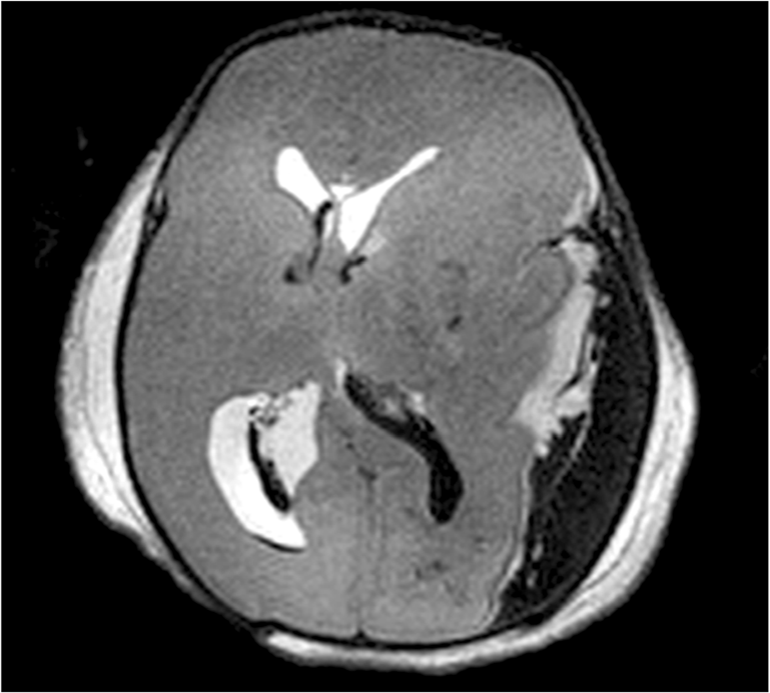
PMMR to document injuries. Post mortem imaging is useful to document the extent of intracranial injury prior to autopsy. In this case, axial T2-weighted PMMR demonstrates a large left parietooccipital subdural hemorrhage, which is causing mass effect on the brain. There is a small amount of intraventricular hemorrhage in addition
